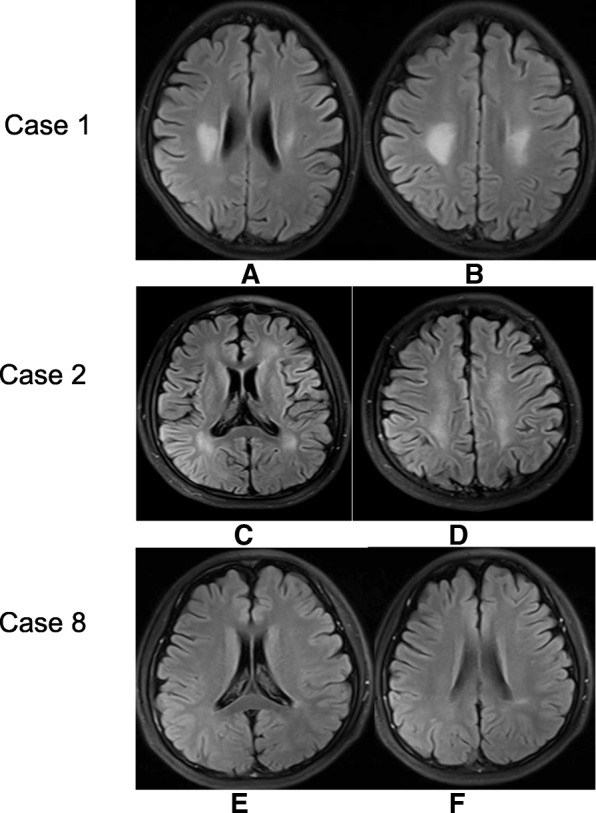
White matter lesions in three cases with late-onset cblC disease. The brain MRI of case 1 in Table 1 showed symmetric patchy lesions in corona radiata (a) and centrum ovale (b). The brain MRI of case 2 in Table 1 showed symmetric patchy lesions in bilateral periventricular white matter, especially in posterior area (c) and corona radiata (d). The MRI of case 8 in Table 1 showed the small lesions in unilateral posterior ventricular area (e, f). The white matter lesions in these three cases presented hyperintensity on fluid attenuated inversion recovery (FLAIR) image
